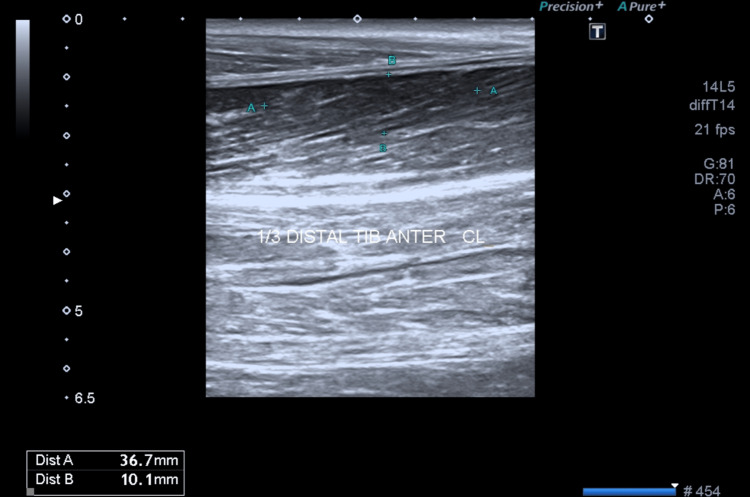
Ultrasound of the left leg showed a rupture of the distal third of the tibialis anterior muscle with an extension of 36 x 10 x 27mm
